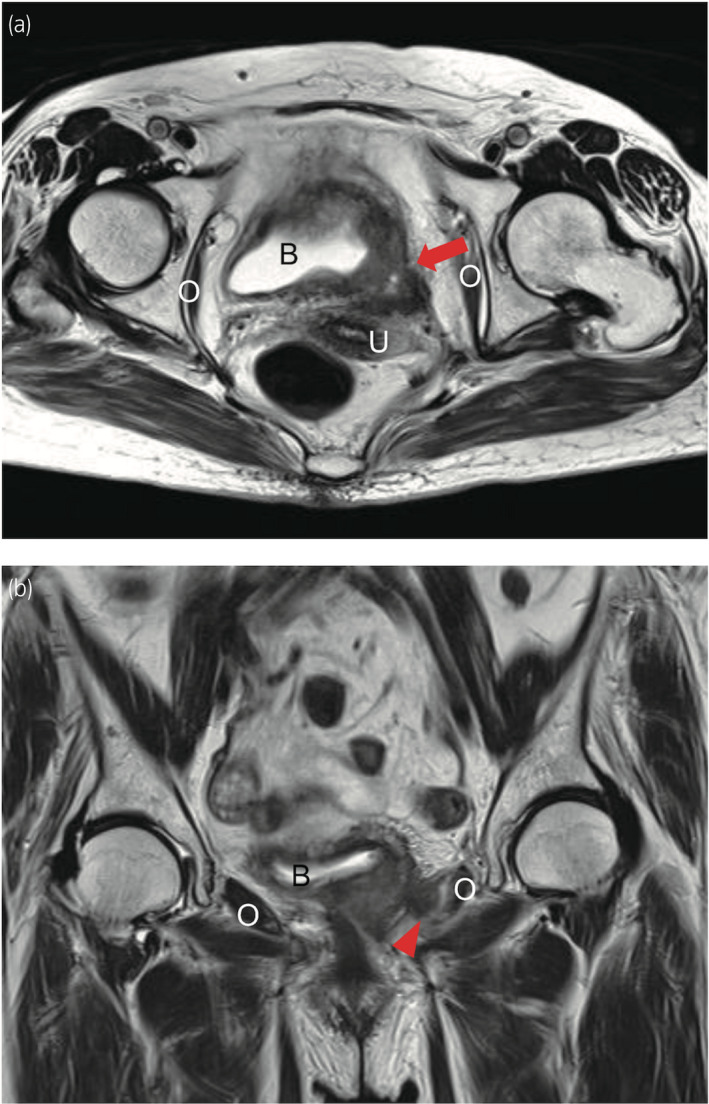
MRI findings of bladder cancer after radiochemotherapy. (a) Axial view: bladder cancer extends to the perivesical tissue (arrow). (b) Coronal view: bladder cancer attaches to the left internal obturator muscle (arrowhead). B, bladder; O, internal obturator muscle; U, uterus.
